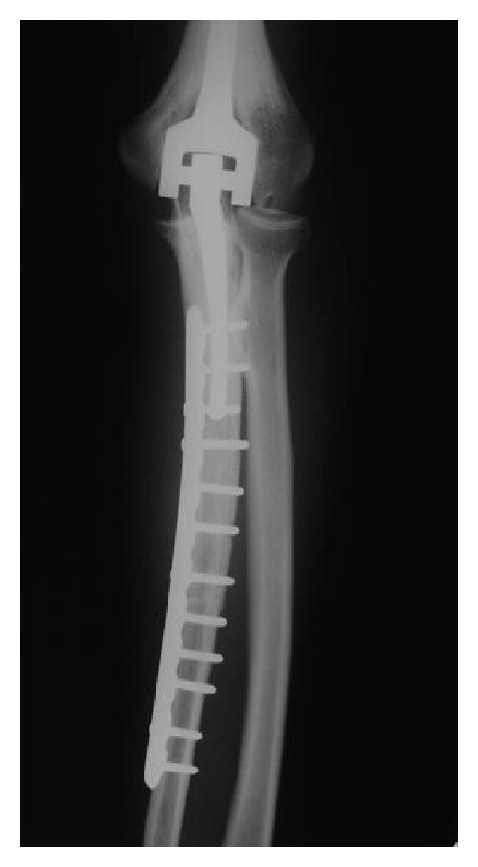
Anteroposterior radiograph of an APC of the elbow after resection of the proximal ulna.
